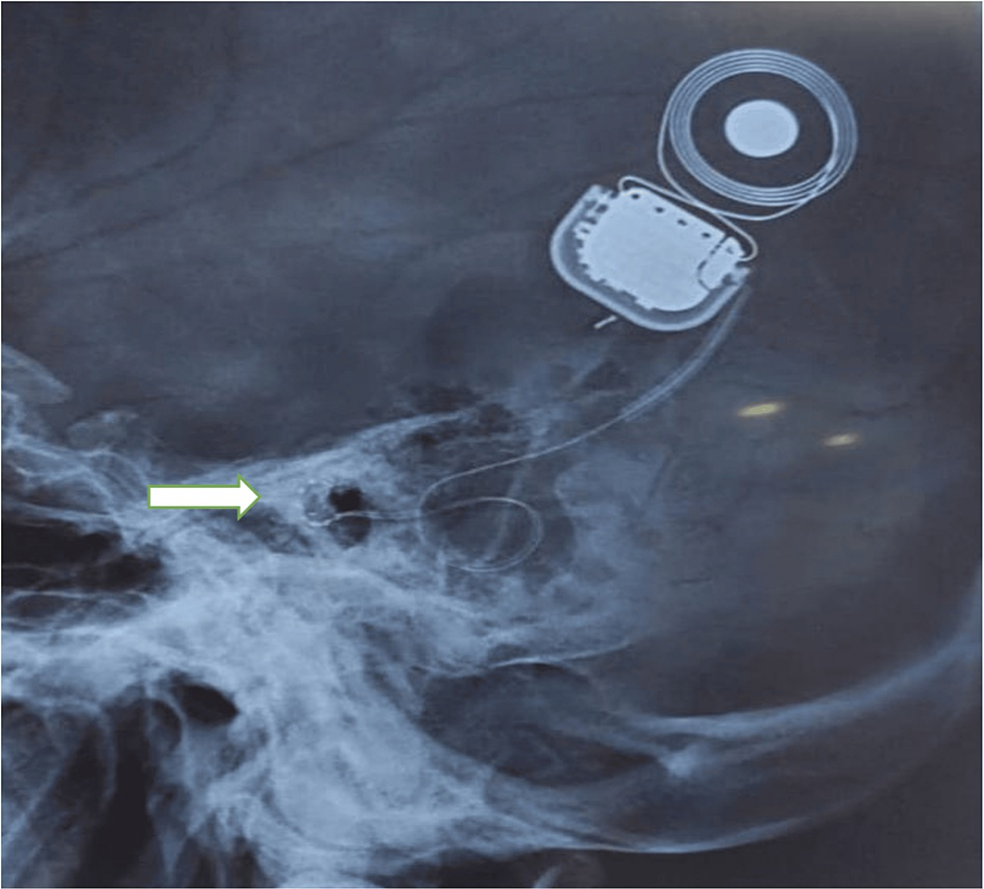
Postoperative X-ray image confirming the presence of electrode in the cochlea (white arrow)
